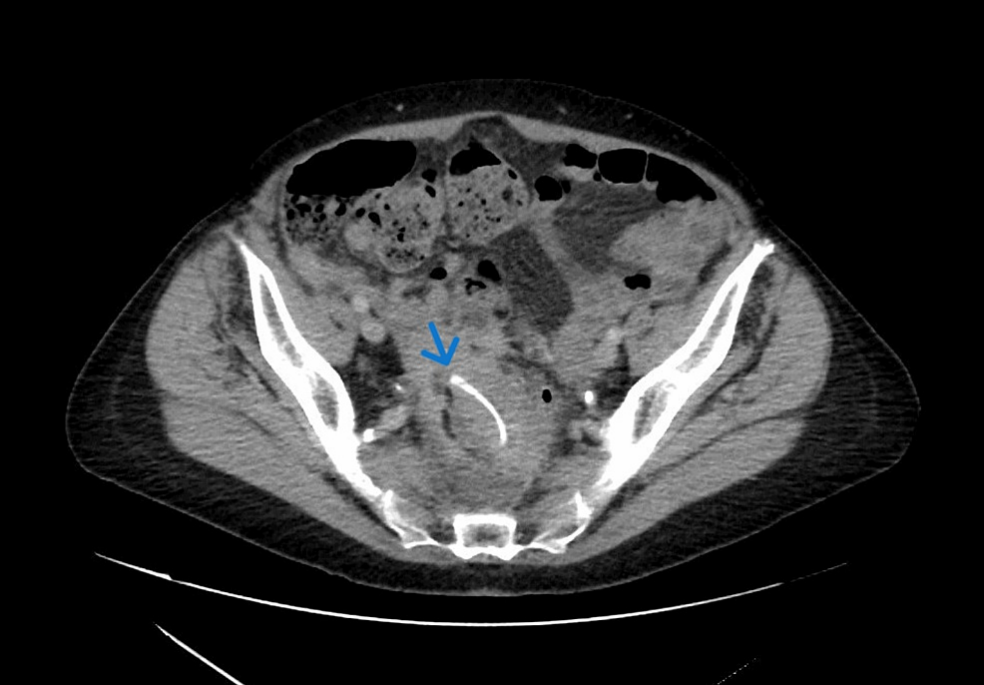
Abdomen and pelvis CT scan demonstrating the chicken bone (the blue arrow). CT: computed tomography.
